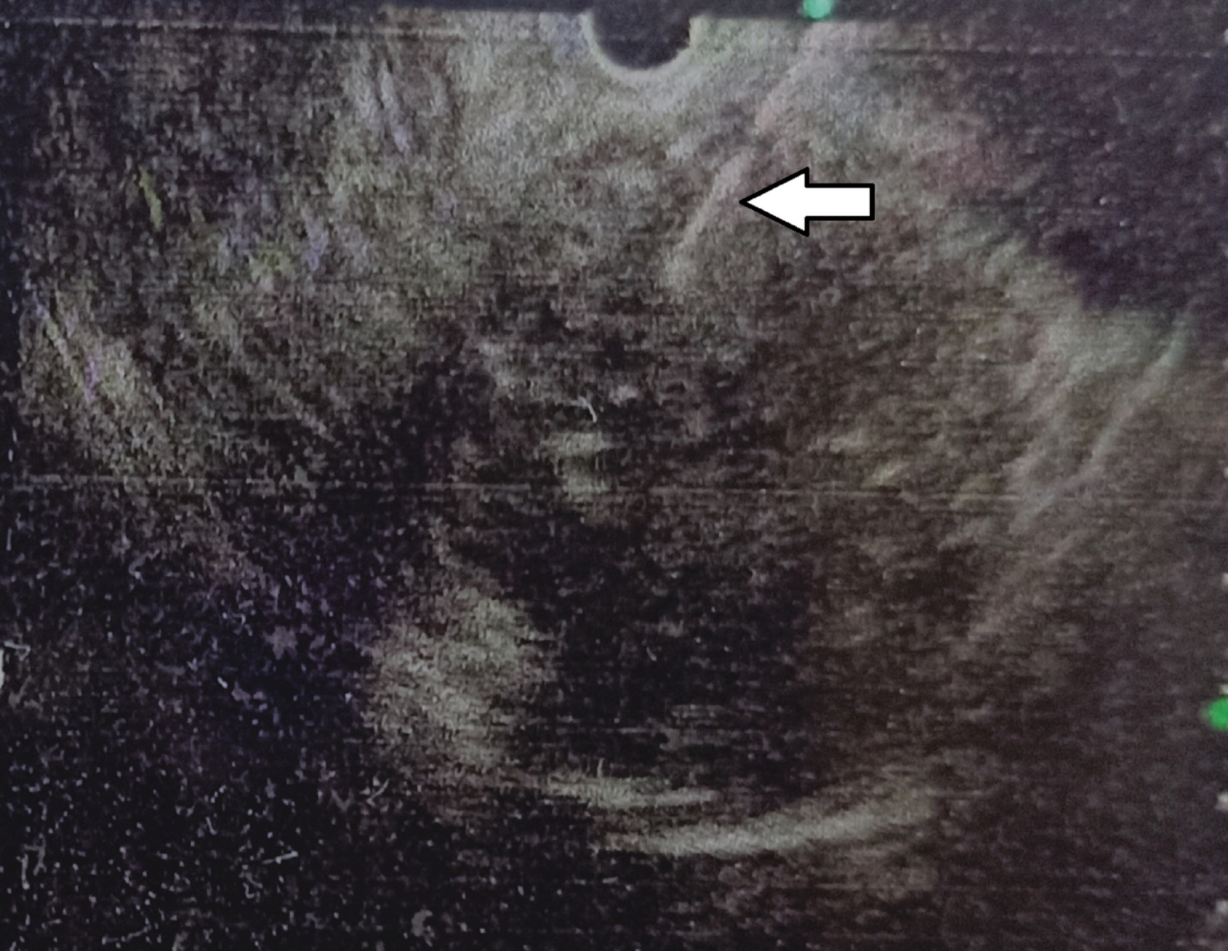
EUS-guided FNAC taken from solid component (marked with arrow) EUS: endoscopic ultrasound, FNAC: fine needle aspiration cytology
